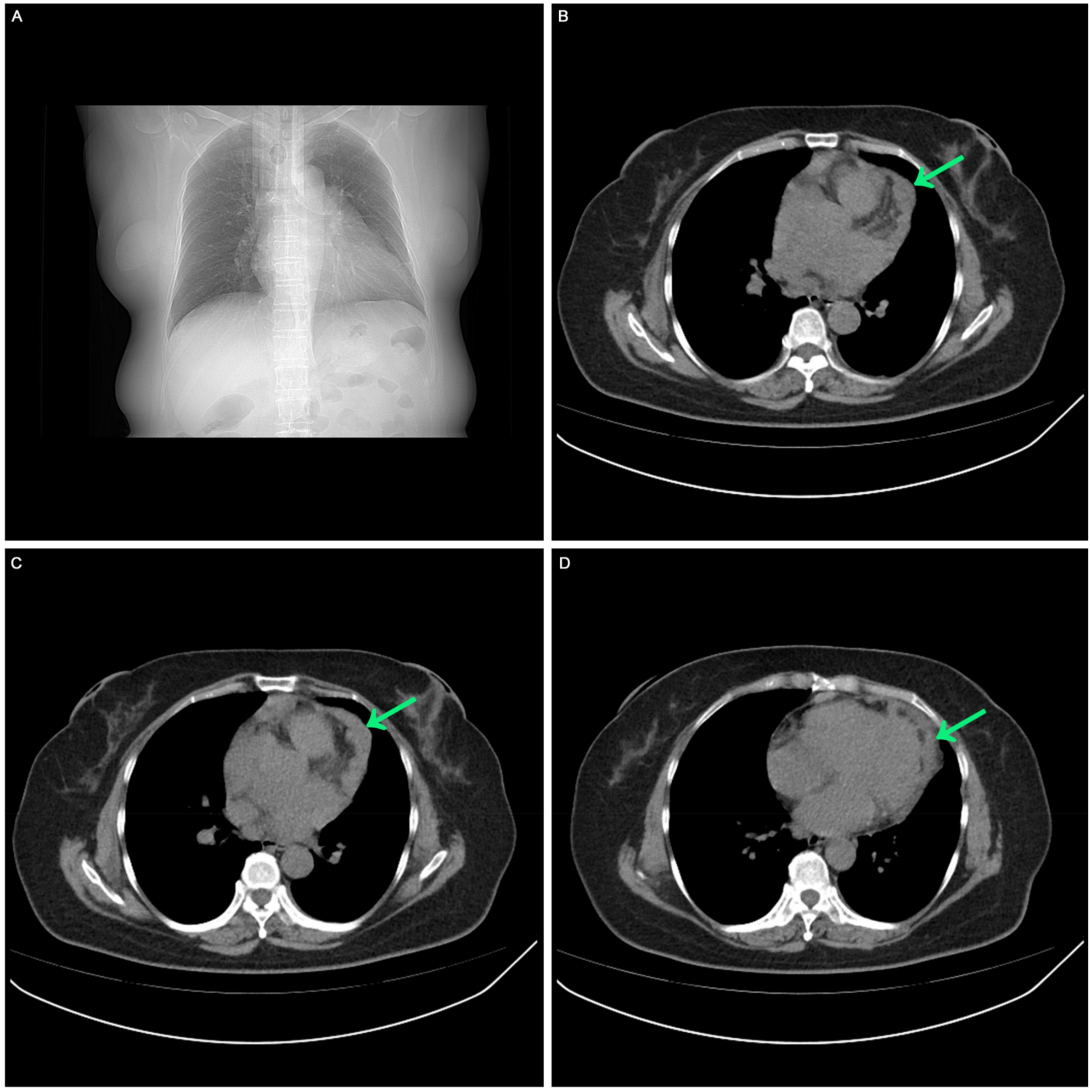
The first visit in May 2023. (A) Chest CT scan showed no pleural effusion, no pericardial effusion. (B, C, D) Chest CT scan showed irregular low-density mass in the left side of the heart.
